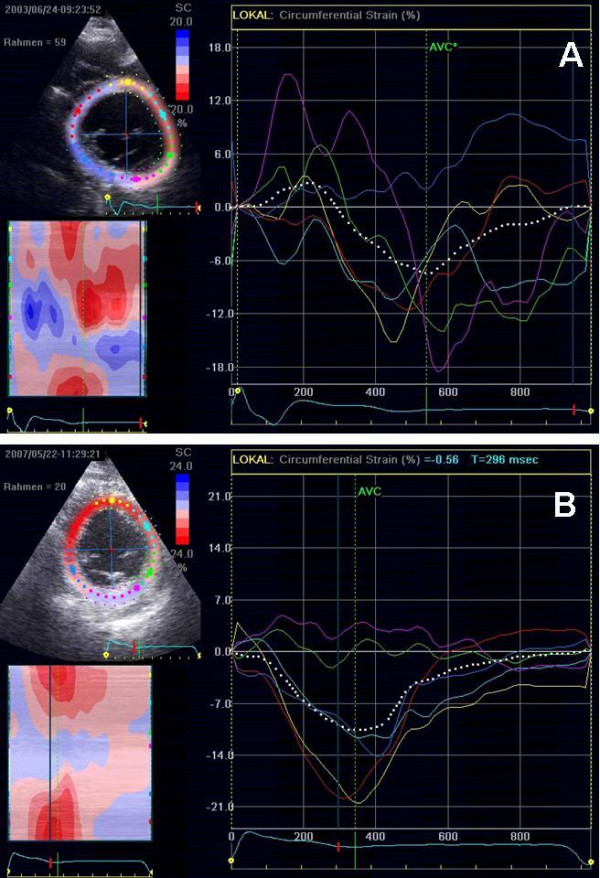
Circumferential 2D strain before (A) and after (B) 4 years of CRT in a responder. Parasternal short axis view at the level of the papillary muscles. Before CRT, there is asynchronous circumferential contraction with postsystolic shortening and passive movement of the inferior and posterior segments, which are scar tissue. After 4 years of CRT, there is increased synchronous contraction, a reduction of postsystolic shortening; the scarred segments (inferior and posterior) show no circumferential contraction.
